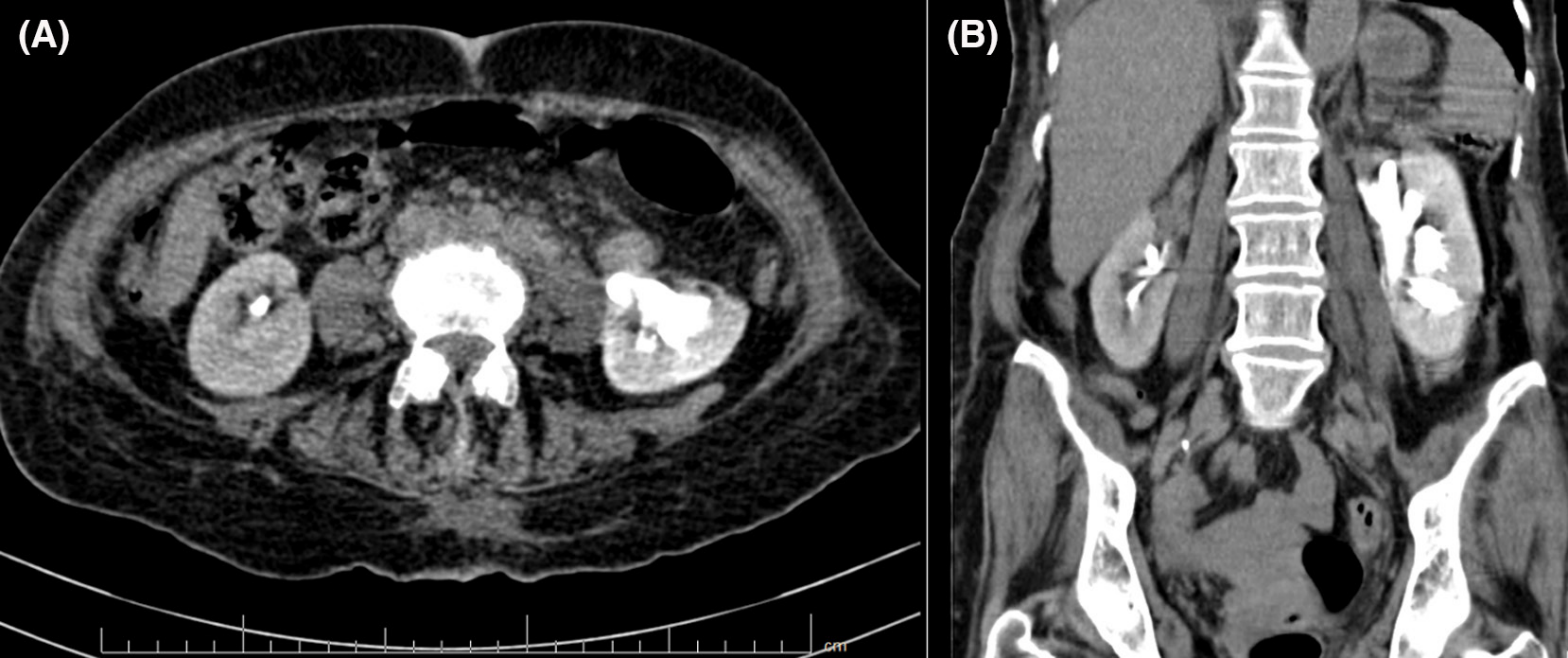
The abdominopelvic CT scan, a few hours after venoplasty, shows a significant accumulation of contrast in the left renal pelvis (compared to the normal excretion of contrast in the right kidney), without any signs of ongoing retroperitoneal hemorrhage or perinephric collection (A: Axial view, B: Coronal view).
